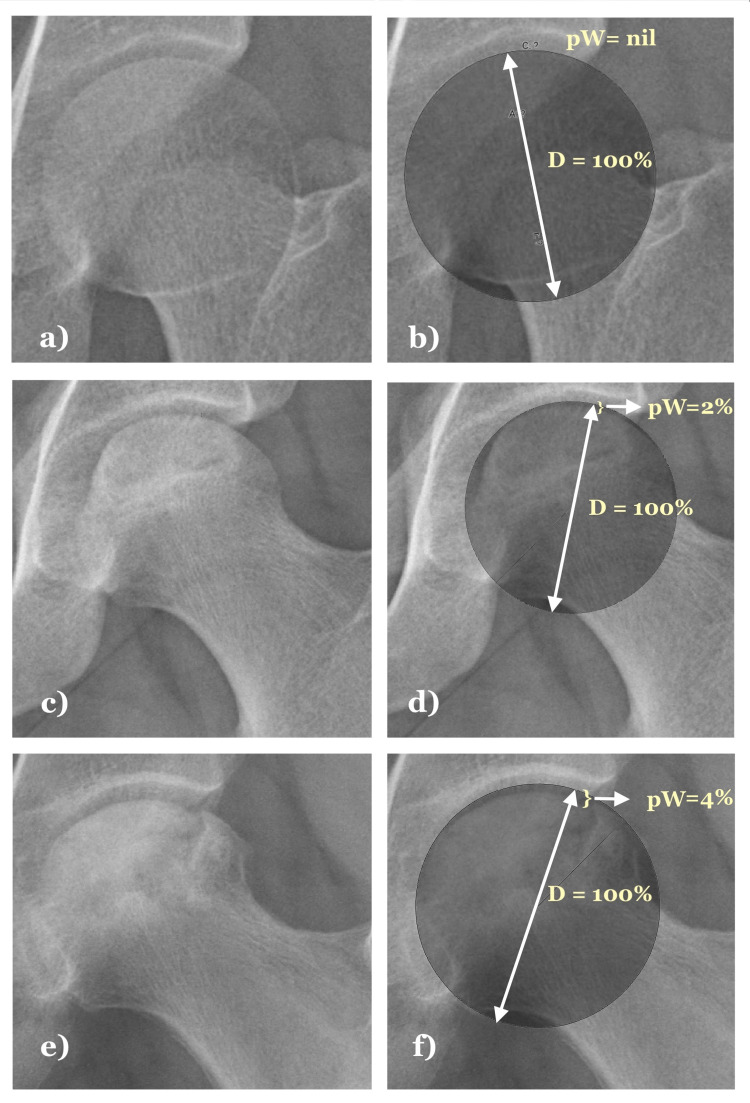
Assessment of femoral head deficiency by superimposing a matching diameter circle in hip AP radiographs. Nil deficiency is seen in a normal femoral head (a, b). Subtle deficiency observed in pre-collapse ONFH affected hip radiograph (c, d). Larger deficiency observed in post-collapse ONFH affected hip radiograph (e, f). ONFH: Osteonecrosis of the femoral head
